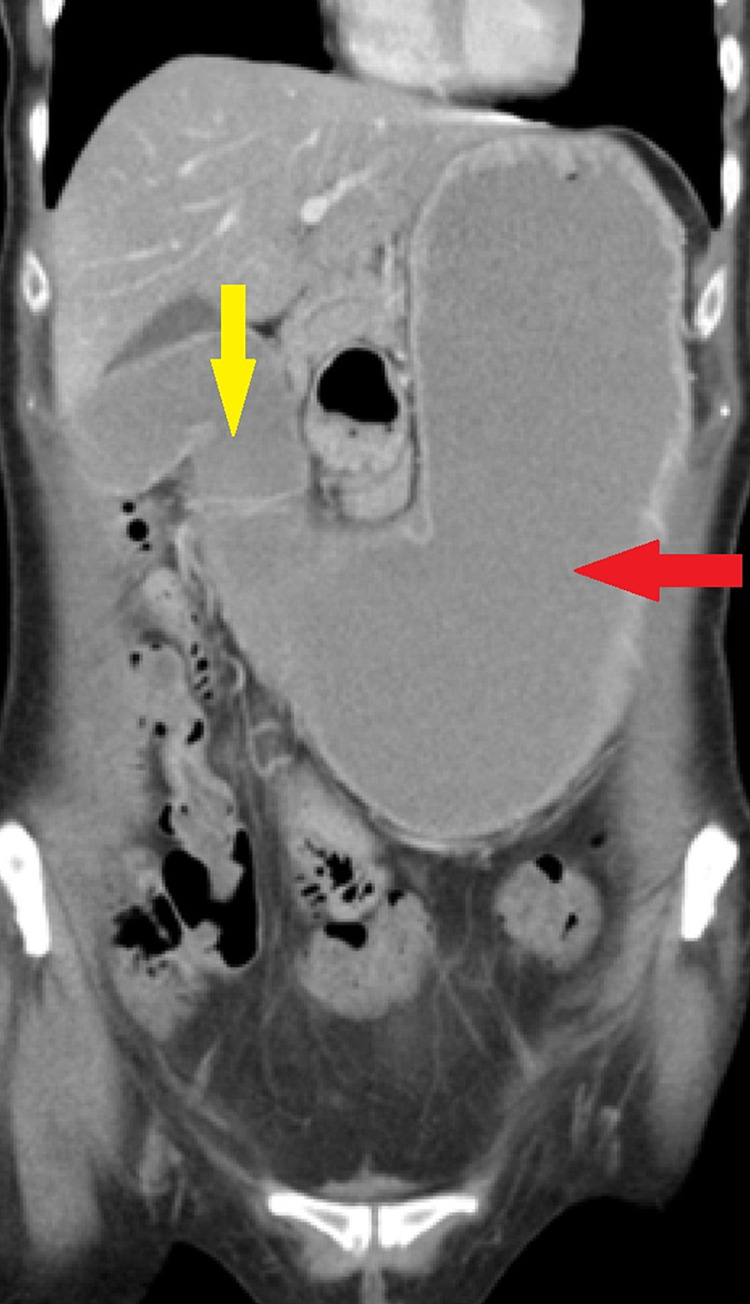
CT scan showing grossly distended stomach (red arrow) with thickening of the pylorus (yellow arrow) and distension of the proximal duodenum.
